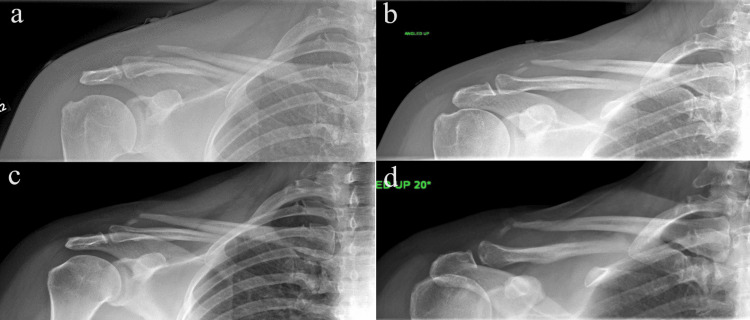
Case 1: Two-week (a and b) and three-month (c and d) follow-up X-ray revealing additional displacement and shortening, with medialization of the lateral clavicle fragment a and c depict the anterior-posterior shoulder X-ray, while b and d show the 20° cephalic tilt shoulder X-ray, highlighting notable medialization and shortening.
